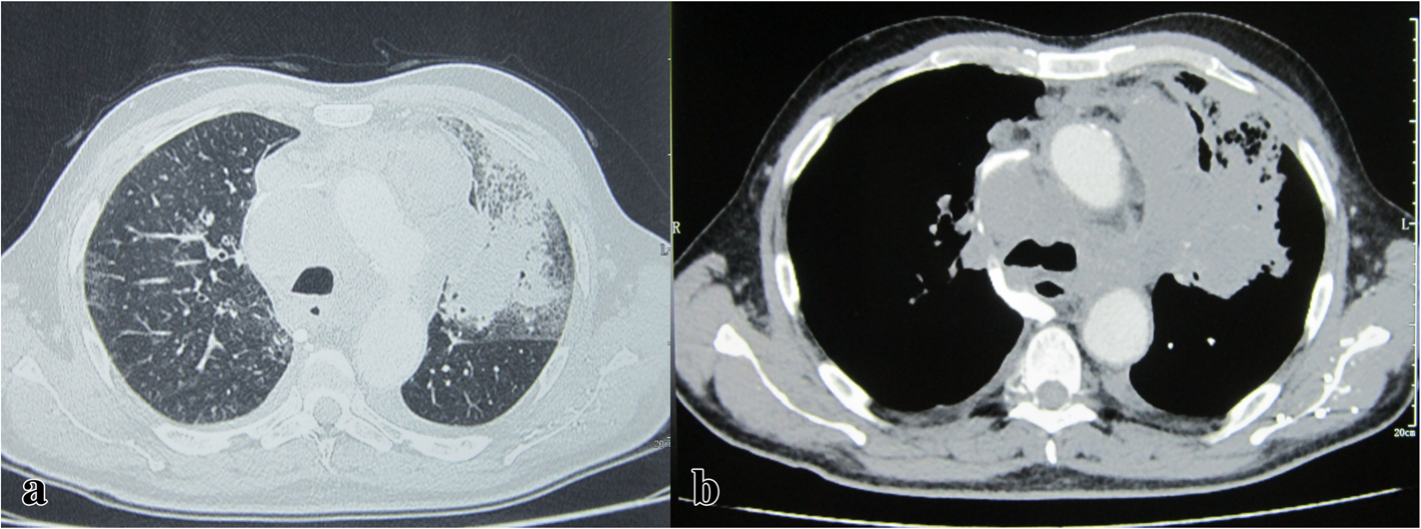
Control chest CT. Extension of the left upper lobe lesion on the parenchymal window (a). Increase in lymph nodes enlargement and superior vena cava obstruction on the mediastinal window (b)
